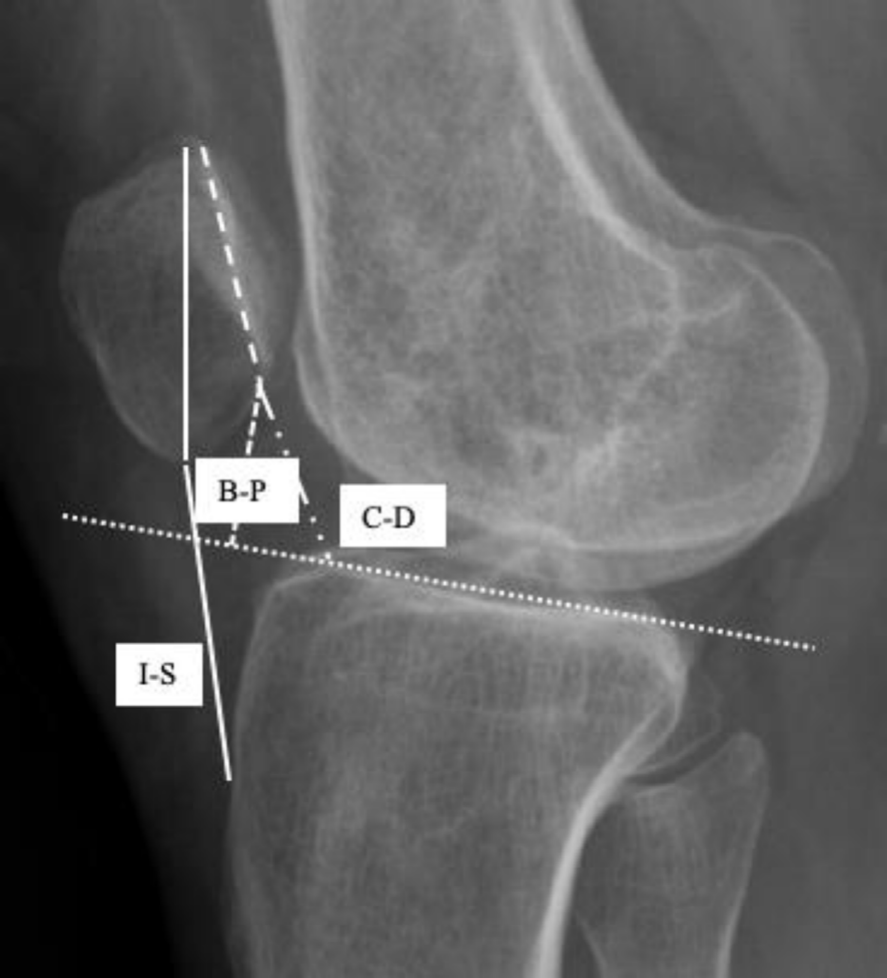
Three common methods for measuring patella alta or patella baja on the radiographs: the Insall-Salvati ratio, the Caton-Deschamps ratio or the Blackburn-Peel ratio.
